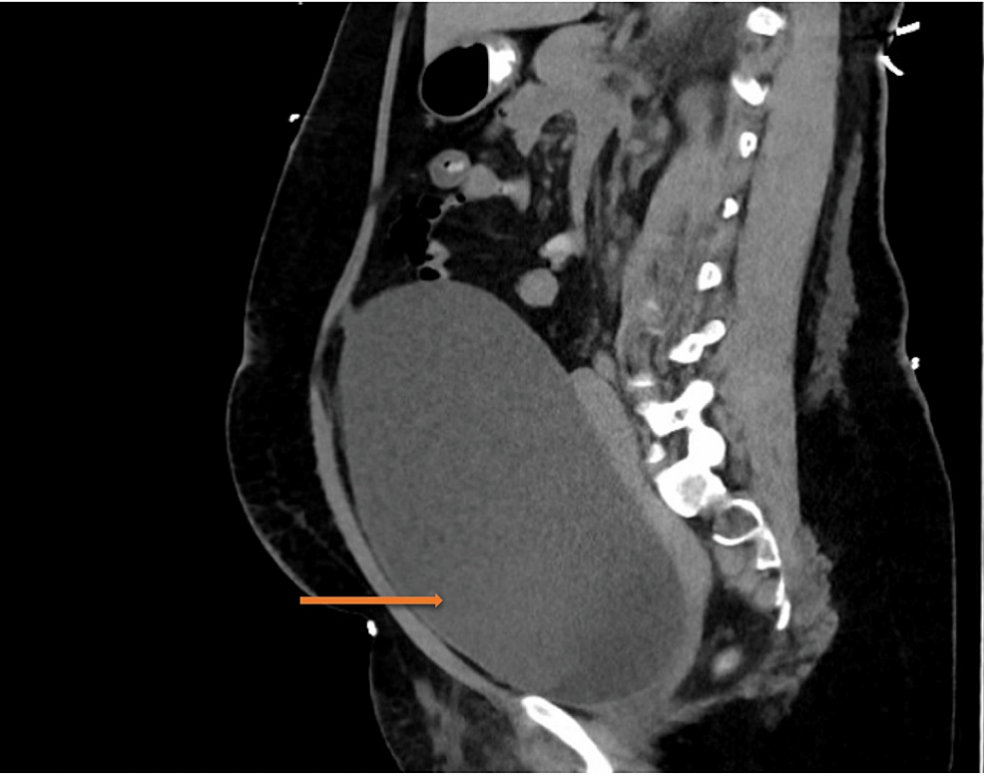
CT scan of the abdomen showing a huge distended bladder (arrow) CT: computed tomography
